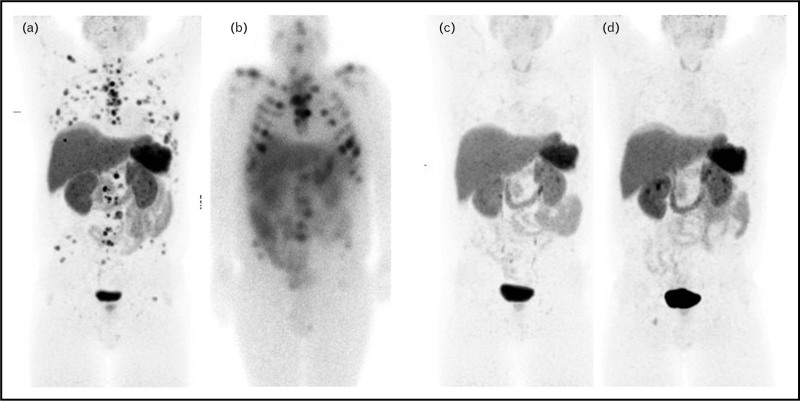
[^68^Ga]dotatate PET showing multiple dotatate-avid skeletal metastases (a). Post [^177^Lu]dotatate therapy SPECT (b), confirms therapeutic targeting. [^68^Ga]dotatate PET after completion of four cycles of [^177^Lu]dotatate therapy (c) shows complete metabolic response. [^68^Ga]dotatate PET (d), 6 months after therapy, confirms a maintained response.
